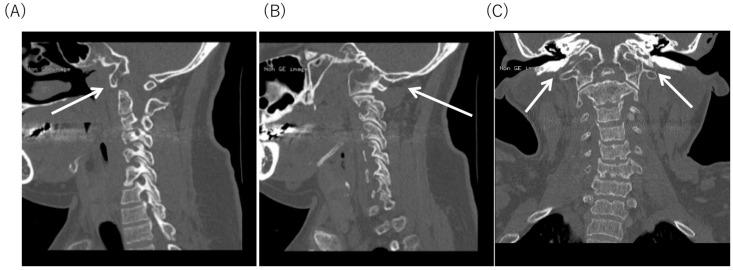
Cervical spine CT at 29 years old. Occipitalization of Atlas is seen. (A) Sagittal section. Occipitalization of the anterior arch of the first cervical vertebra and the lower surface of the occipital bone at the part indicated by the white arrow. (B) sagittal section and (C) coronal section. Occipitalzation of the lateral mass of the first cervical vertebra and the lower surface of the occipital bone are fused at the area indicated by the white arrow.
